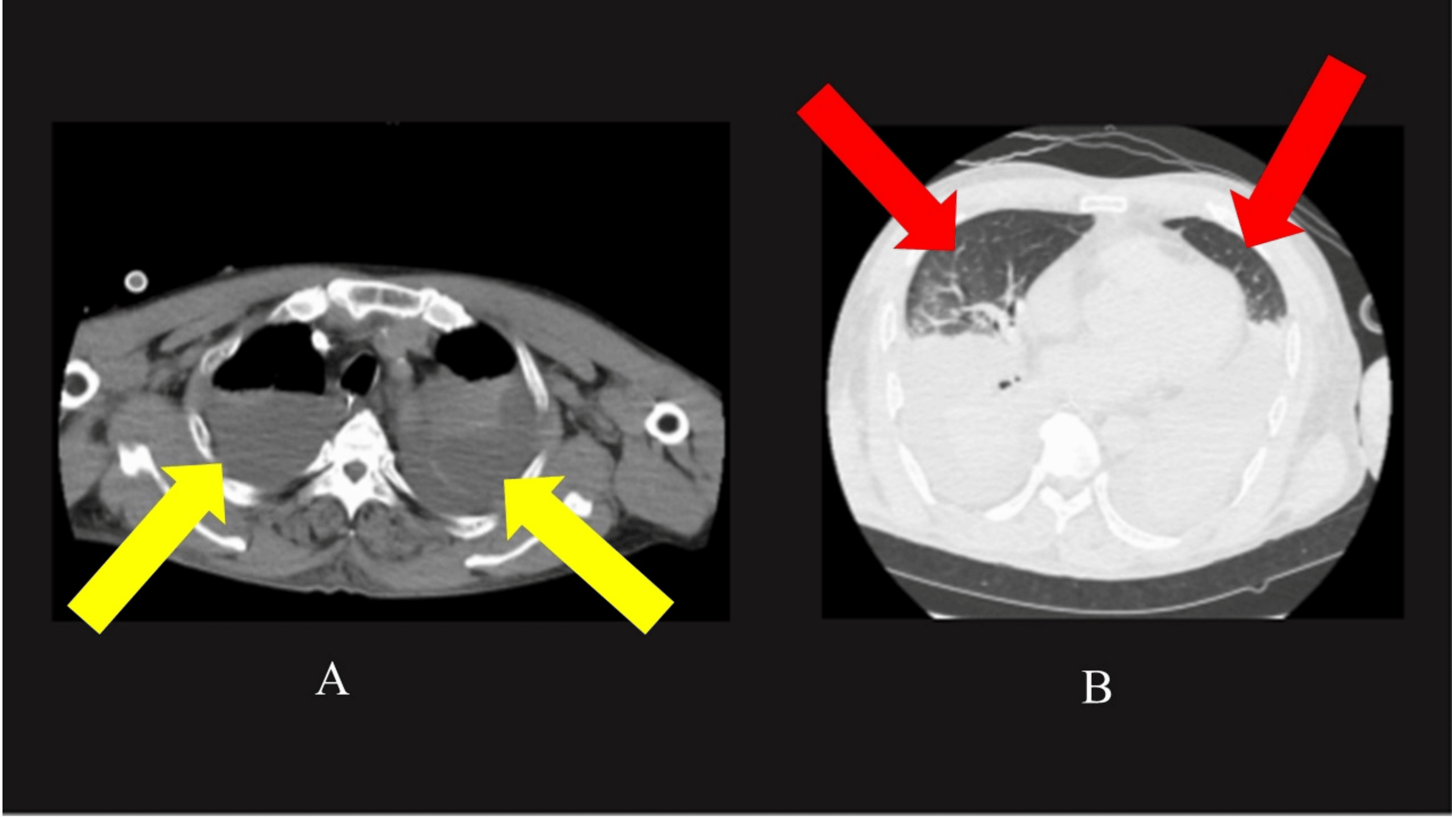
Plain CT of the lung taken after the introduction of ECMO A: In the mediastinal window, bilateral pleural effusion and atelectasis (yellow arrows) are observed. B: In the lung window, only a small portion of normal lung tissue is visible (red arrow). ECMO, extracorporeal membrane oxygenation; CT, computed tomography
